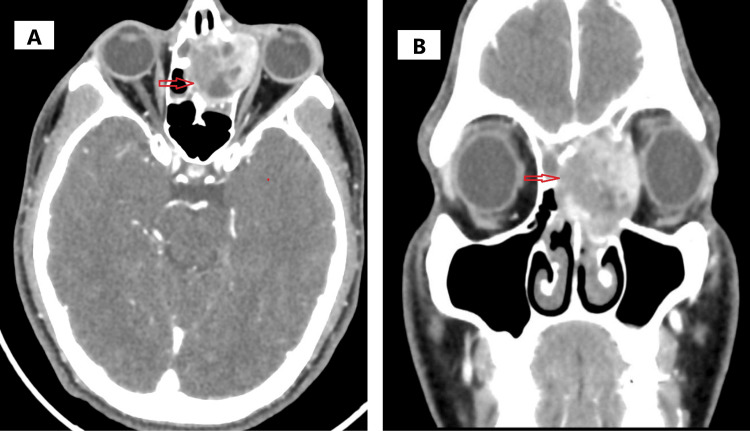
Radiology images A&B: Contrast-enhanced CT scan showing a well-defined lobulated lesion in the left ethmoid sinus with orbital extension.
